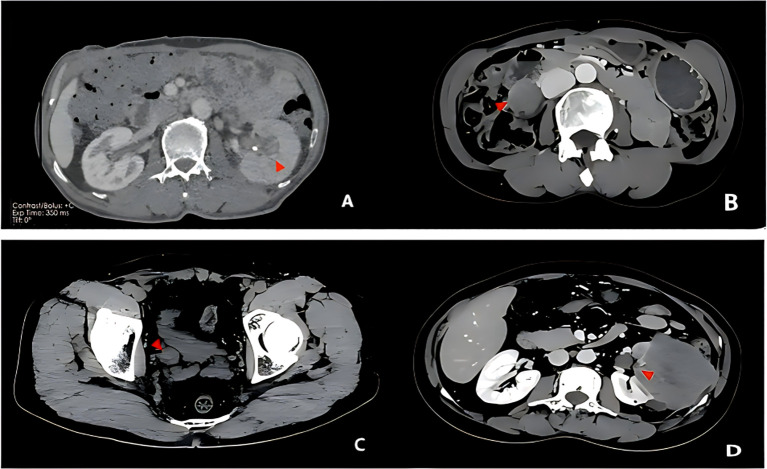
Enhanced CT images of Case 1 (A), Case 2 (B), Case 3 (C), and Case 4 (D).
